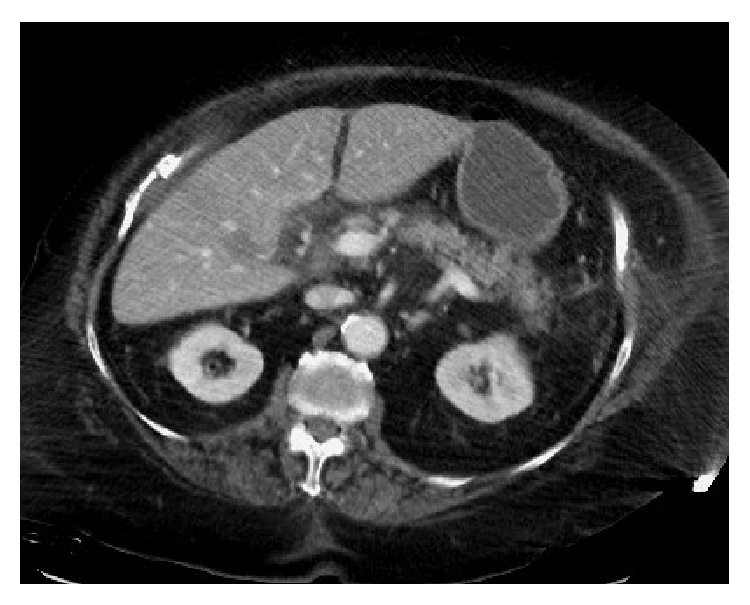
Axial CT image demonstrating inflammation of the head and body of the pancreas.
